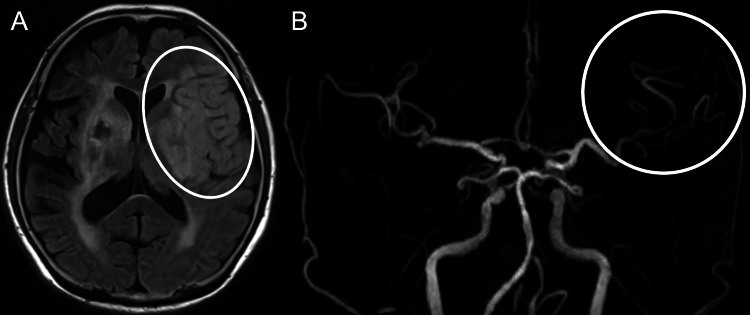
MR images after second mechanical thrombectomy (A) MRI FLAIR sequence showing an ischemic lesion in the left frontal lobe (oval). (B) MRA revealing partial recanalization of the left MCA (circle). MRI: magnetic resonance imaging; FLAIR: fluid-attenuated inversion recovery; MRA: magnetic resonance angiography; MCA: middle cerebral artery
